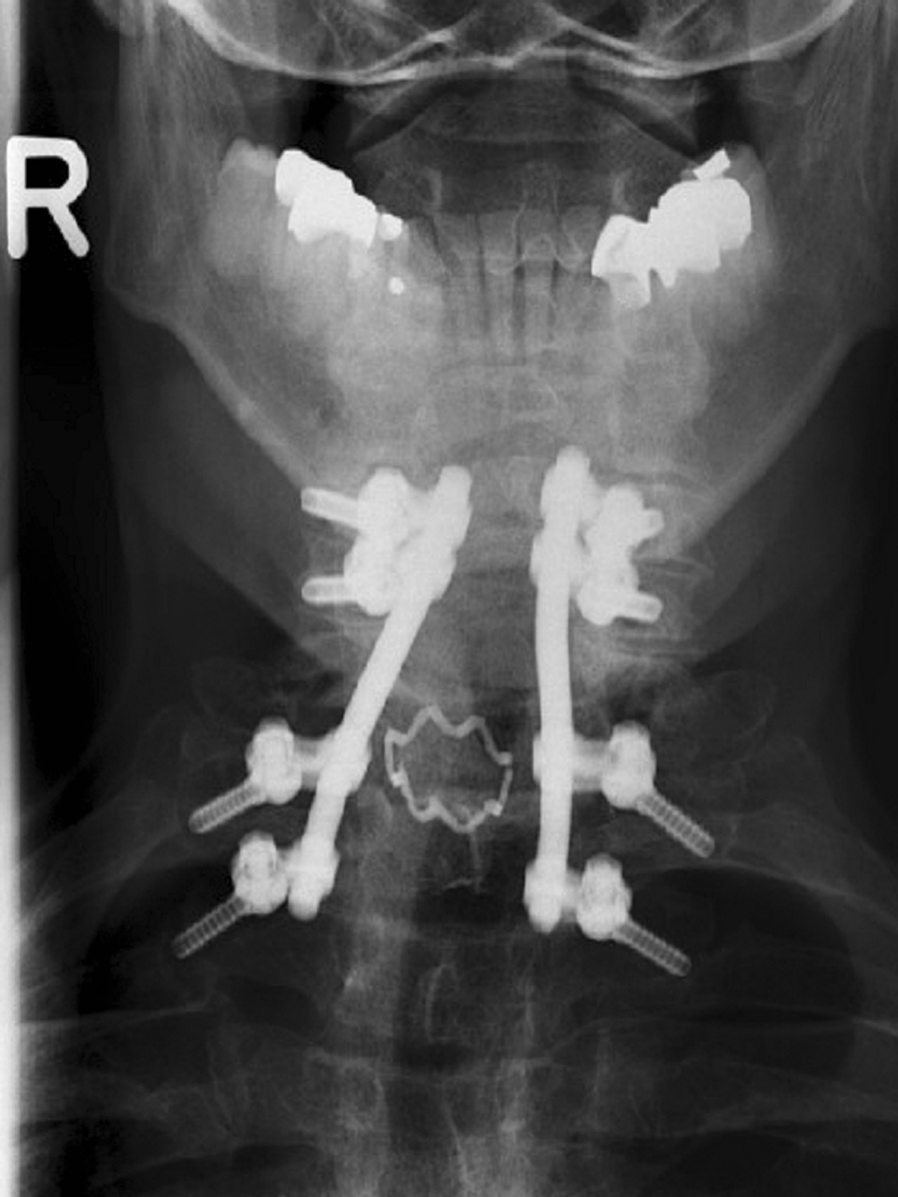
Anterior-posterior radiograph of the cervical spine shows the treatment of the spondylolisthesis at C7-T1; the lower screws were fixed bicortically into the transverse processes of T2 and T3.
